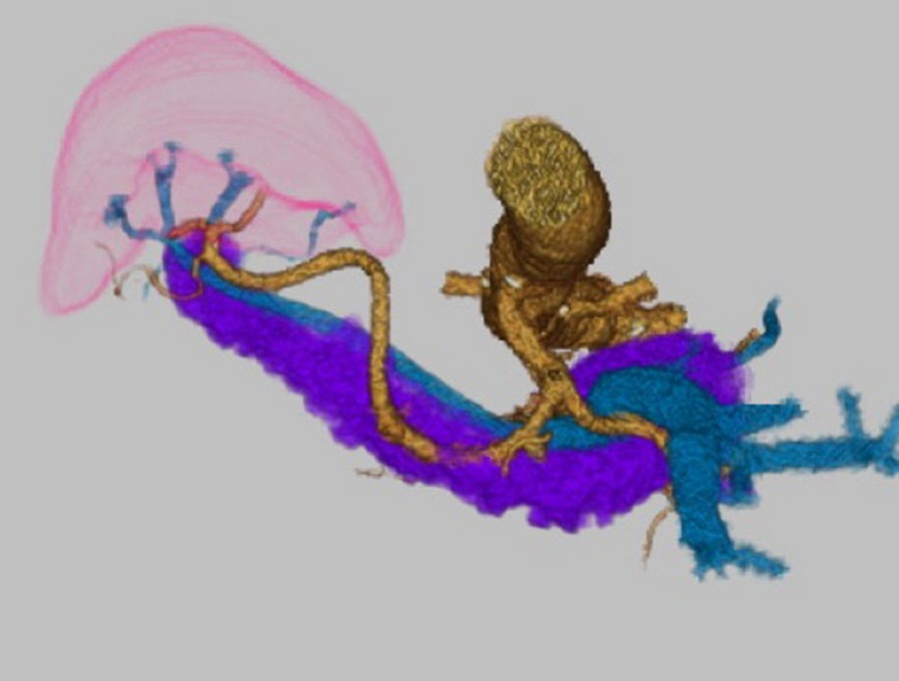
Three-dimensional reconstruction of an abdominal computed tomography angiogram preoperatively
